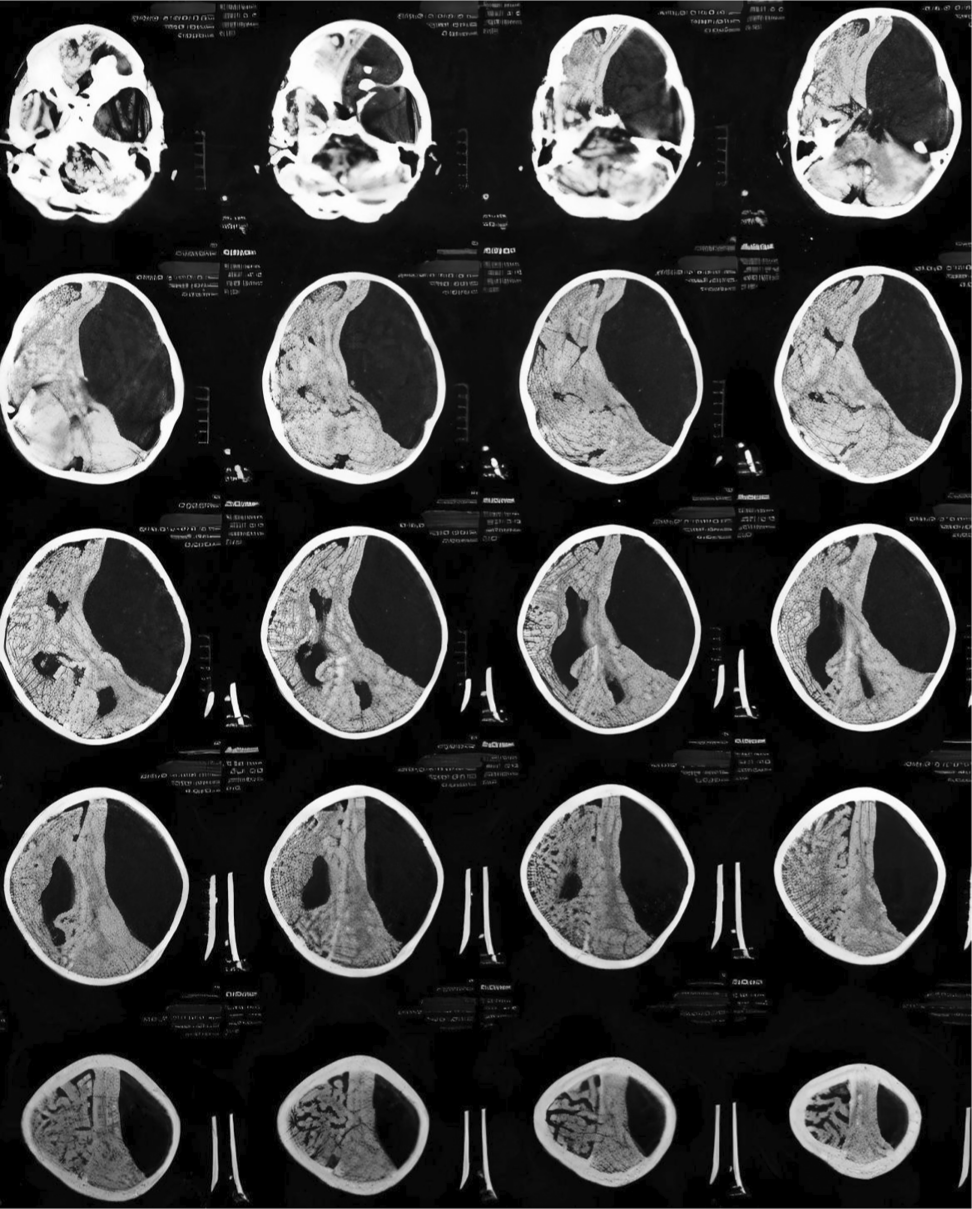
Non‐contrast computed tomography (CT) brain showing left sided large Frontotemporoparietal (Galassi Type III) Arachnoid cyst. A midline shift secondary to the mass effect of the cyst can be noted.
